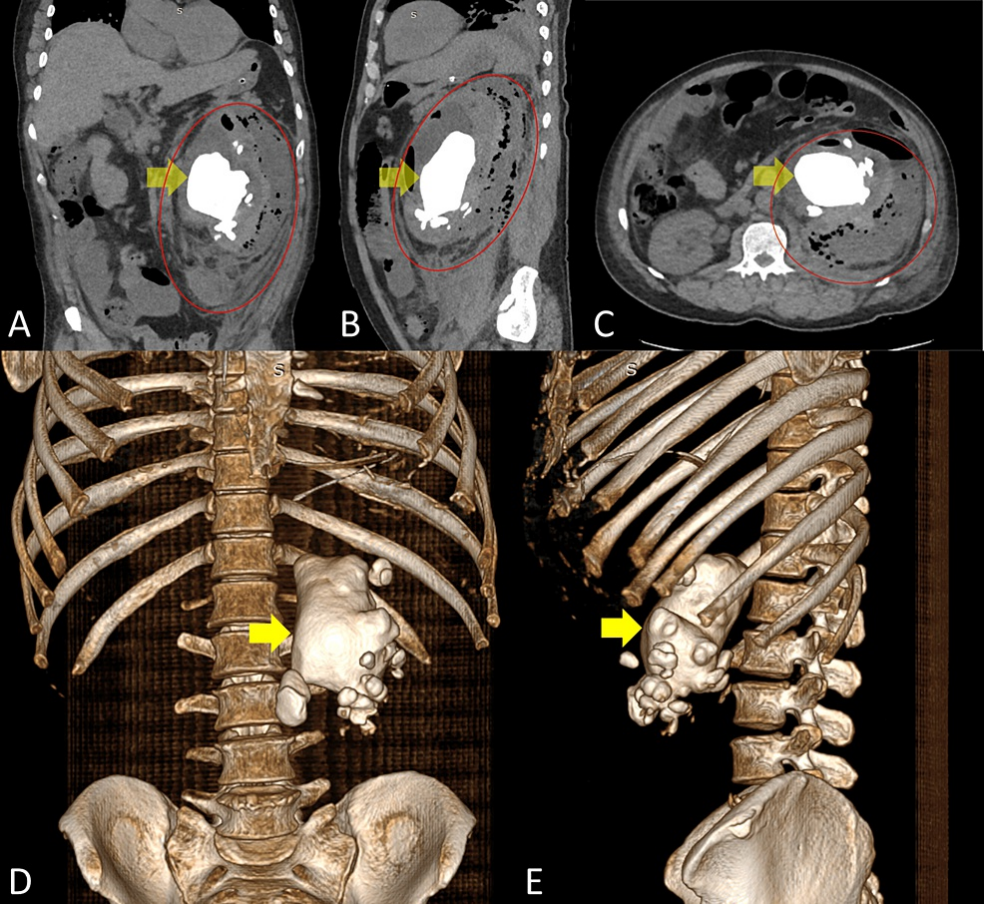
Non-enhanced abdominopelvic CT scan showing staghorn nephrolithiasis with a renal abscess. The yellow arrow points to a hyperdense region indicative of staghorn lithiasis, while the red circle encompasses the hypodense area where the abscess was identified. A: Coronal section. B: Sagittal section. C: Axial section. D: Three-dimensional reconstruction, anterior view. E: Three-dimensional reconstruction, left lateral view.
